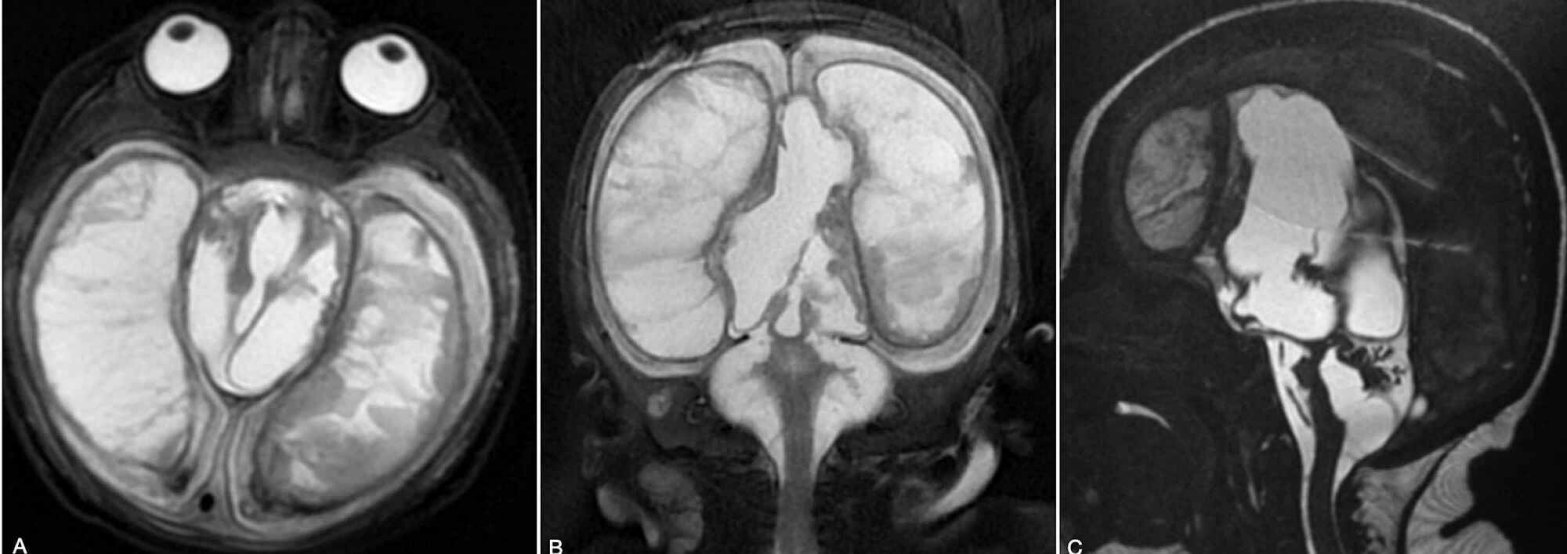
Dysmorphic brain expansion MRI after one month of endoscopy. Axial T2-weighted MRI showing brain expansion (A). Trabeculated imaging inside the brain (B). Improvement of compression of the posterior fossa (C).
